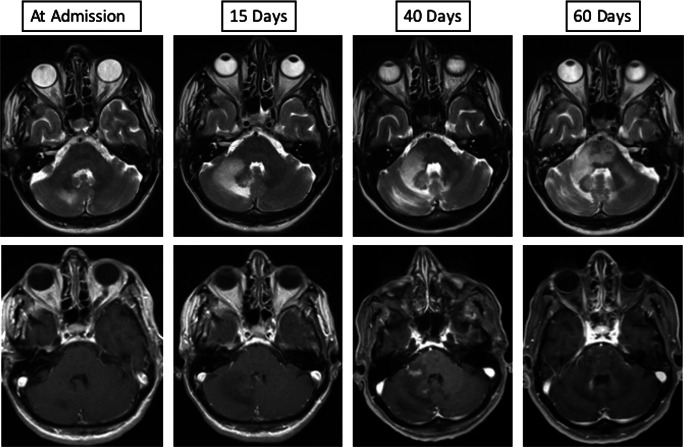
Cerebral magnetic resonance imaging (cMRI) findings. Upper row shows progressive hyperintensity of the infratentorial white matter on axial T2 images representing increase of parenchymal edema (left to right images). Lower row shows axial T1 contrast-enhanced images of the first enhancement of the infratentorial white matter at day 40 after initial imaging that represents the switch from progressive multifocal leukoencephalopathy (PML) to PML immune reconstitution inflammatory syndrome (IRIS) as a radiological finding
